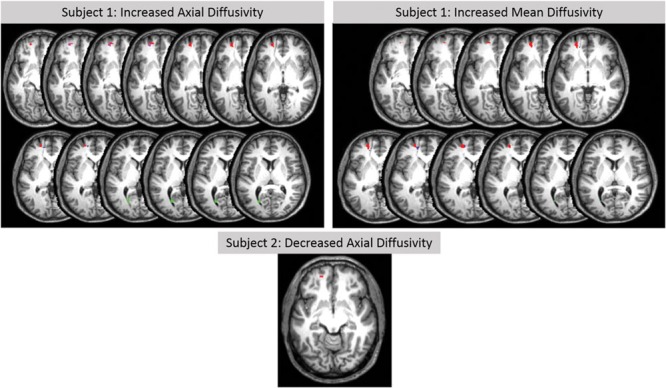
T_1_-weighted axial MRI images of DTI-based analysis in subjects 1 and 2. Increases in AD (left top images) and in MD (right top images) are shown in subject 1 in the left superior frontal gyrus (red), left middle frontal gyrus (magenta), left anterior cingulate gyrus (blue), and left posterior cingulate gyrus (green). A decrease in AD (medial bottom image) is shown in subject 2 in the left superior frontal gyrus (red; decreased MA in the same ROI is not shown). Significant changes (small volume correction, *p* < 0.05 corrected) are relative to a healthy control subjects.
